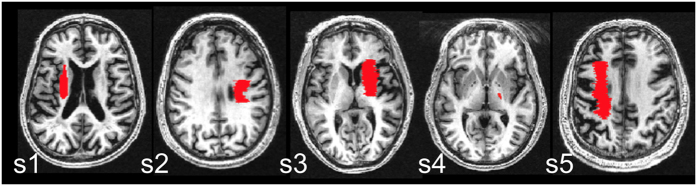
Lesion location. Lesions are shown overlaid on each subject’s T1-weighted image. The slice with the maximal lesion size is shown for each subject (S).
